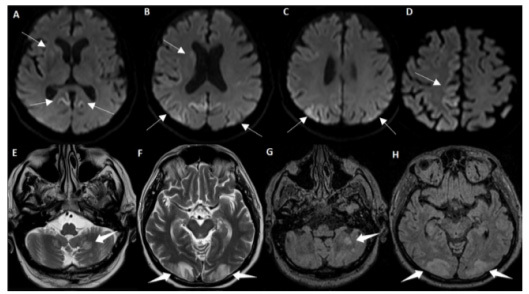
Magnetic resonance imaging features of sporadic Creutzfeld-Jakob disease and posterior reversible encephalopathy syndrome. (A, B, C and D) Initial diffusion-weighted images revealing bilateral hyperintensities in parietal areas, mostly on the right, and hyperintensities in cingulate gyrus. The same findings apply albeit to a lesser extent in the right basal ganglia (caudate and putamen, white arrows). (E, F, G and H) T2WI and T2-fluid-attenuated inversion-recovery reveals new cortico-subcortical hyperintensities in parietal and occipital areas and cerebellum, compatible with vasogenic edema, highly suggestive of PRES (thick white arrows). Previously described alterations remain the same. T1W1 does not reveal contrast enhancement.
